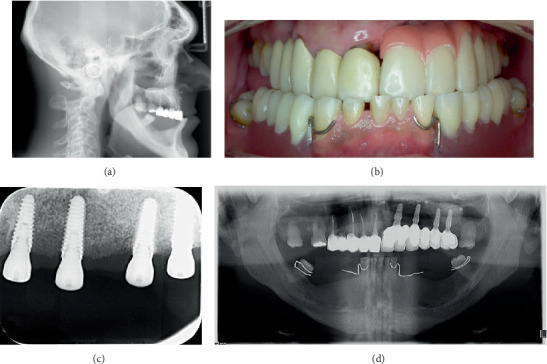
(a) Cephalometric radiograph made before implant placement. (b) Frontal intraoral view with the restorations. (c) Retroalveolar X-ray at implant uncovery. (d) Orthopantomography with the restorations.
